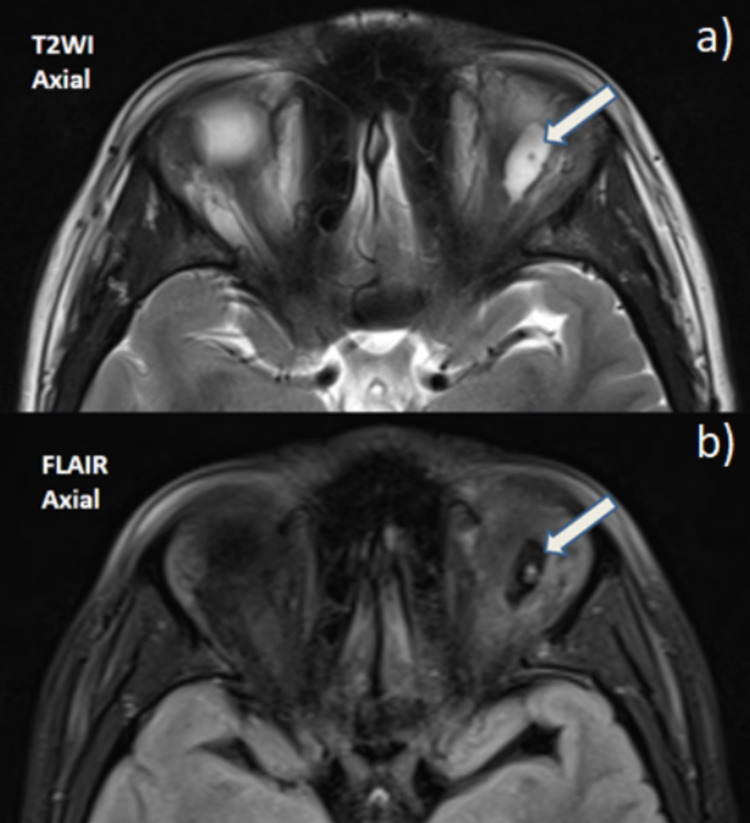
(a) On axial T2WI a focal hyperintense lesion is noted within the superior rectus muscle with a tiny eccentric hypointense mural nodule. (b) On axial FLAIR sequence, the hyperintensity observed on T2WI was suppressed.
